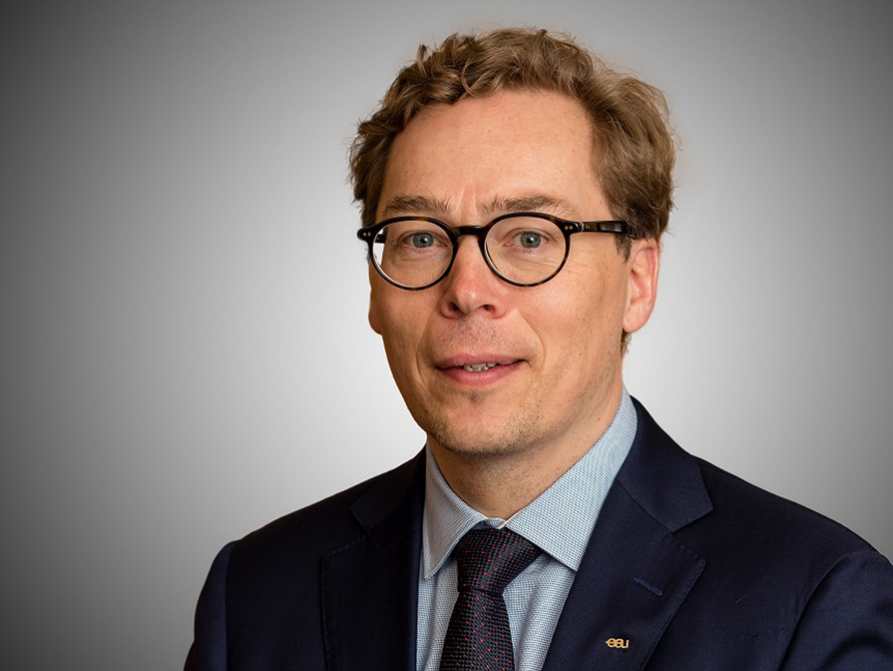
J.P. Michiel Sedelaar. J.P. Michiel Sedelaar is the deputy-chief of the department of Urology at Radboud University Medical Center, Nijmegen, the Netherlands. He finished his urology training in 2007 and in 2008–2009 he was a post-doc researcher at the Johns Hopkins Medical Institutes in Baltimore, MD, USA. His focus during that time was the development of smart-drugs for detection and treatment of prostate cancer and steroidogenesis of prostate cancer. Since 2010, he returned to Nijmegen for an onco-urology staff position. His research achievements are mainly on imaging studies for prostate cancer (use of MRI, MRI-guided treatments) and basic research on the androgen receptor in prostate cancer. He has national and international board functions; among others, he is the chair of the EAU-Young Urology Office
